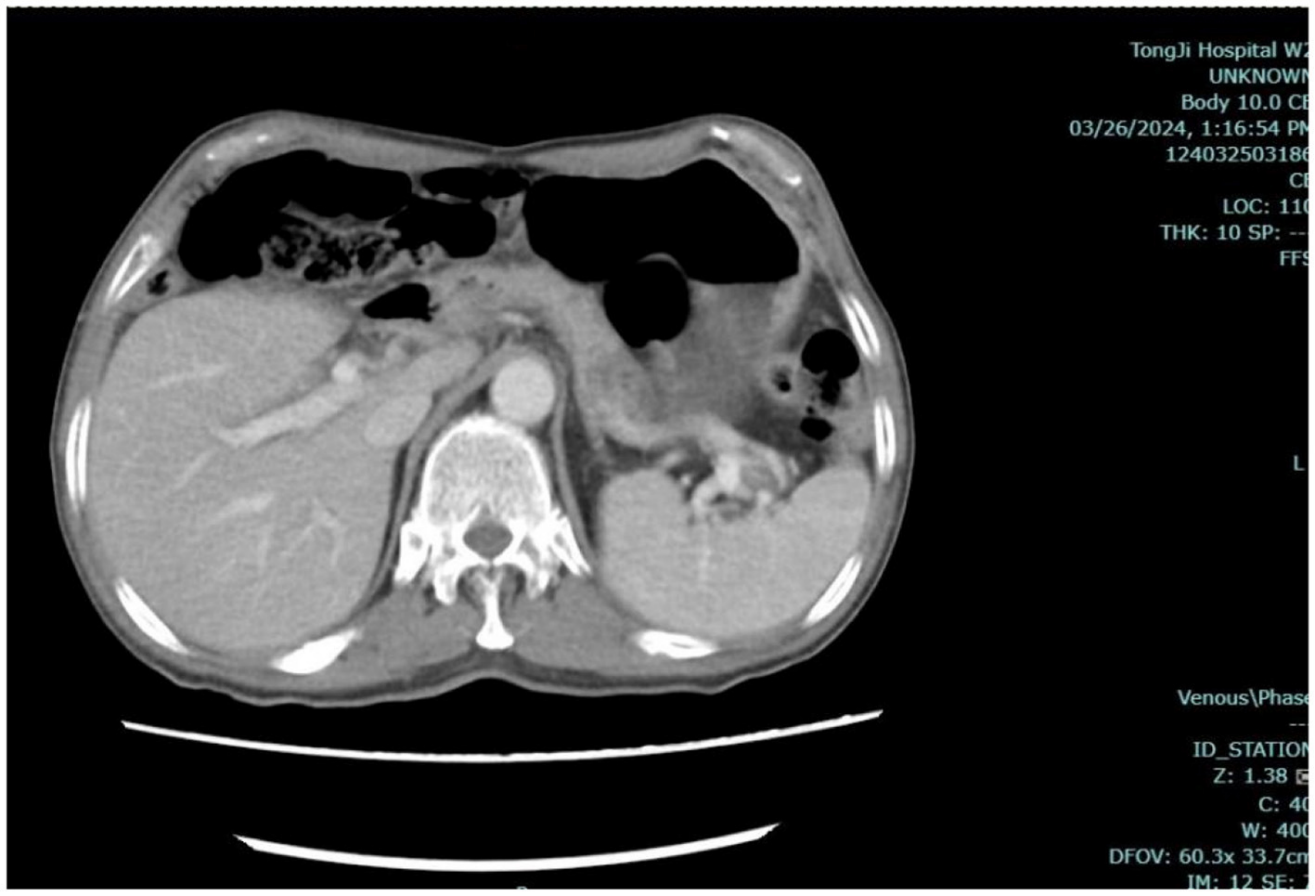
Abdominal CT. Enhanced computed tomography (CT) of the entire abdomen indicated a elongated sigmoid colon, with no obvious signs of obstruction or space-occupying lesions.
